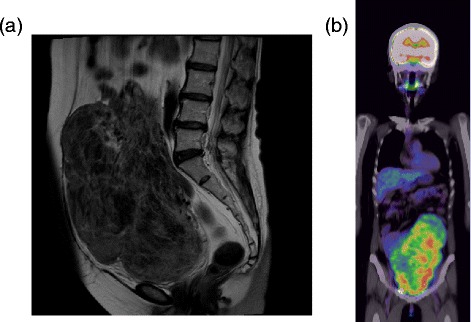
Radiological images. (a) Pelvic magnetic resonance imaging, T2-enhanced sagittal section, showing an irregularly enlarged uterine tumor. (b) Positron emission tomography-computed tomography showing strong uptake associated with the uterine tumor.
